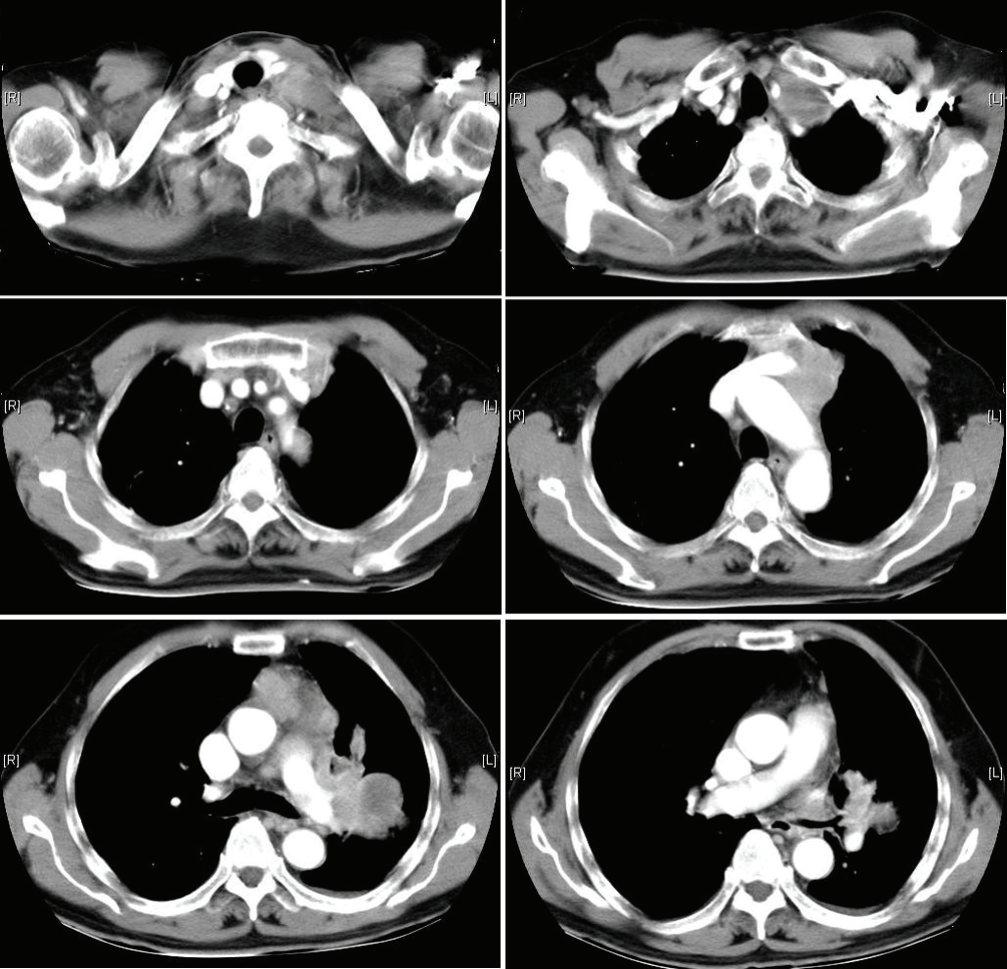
Chest CT (with contrast) demonstrates a 4.5 cm soft tissue mass with heterogenous enhance at left lower lobe with lymphadenopthy at left hilum, left paraaortic, anterior mediastinum and left upper paratracheal site (lower and middle panels). The lymphadenopathy extends to left lower neck and causes obstruction of left upper lobe bronchus, right side displacement of trachea, thyroid gland. Left carotid artery and internal jugular vein are compressed and the latter is not opacified by contrast medium.
